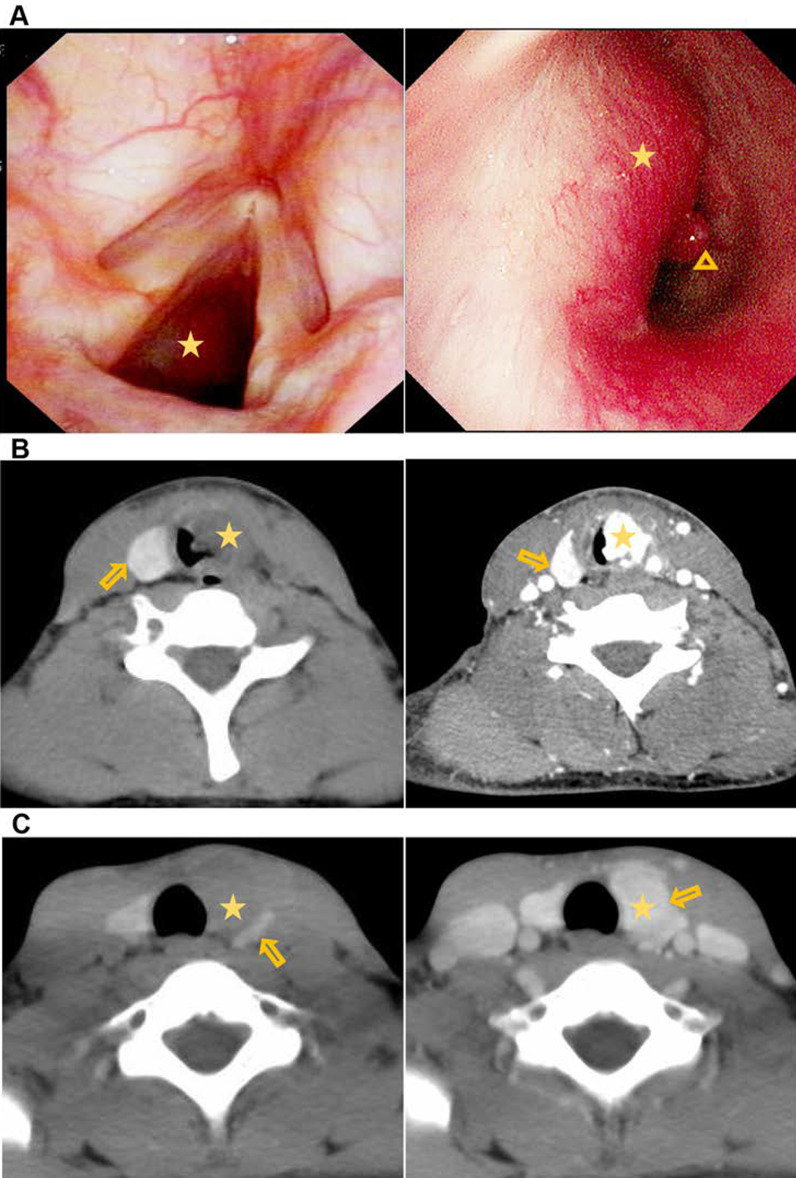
Preoperative imaging of the new reported case. A the smooth and sessile vascular tumor (labeled with asterisk) was seen in the subglottis and vascular proliferation in a pseudo angiomatosus (labeled with triangle) was captured on the tumor surface by flexible fiberoptic bronchoscope (the right image is a close view of the left image). B CT scans with plain (the left) and contrast-enhanced (the right) image showing the intraluminal and extraluminal involvement of left subglottic larynx (labeled with asterisk). The right lobe of the thyroid (labeled with arrow) was present while the left lobe and isthmus of thyroid were absent. C the demarcation line was not clearly recognized between the tumor (labeled with asterisk) and the thyroid (labeled with arrow) by plain CT scan (the left) and contrast-enhanced CT scan (the right) before thyroidectomy (7 years prior to this study)
